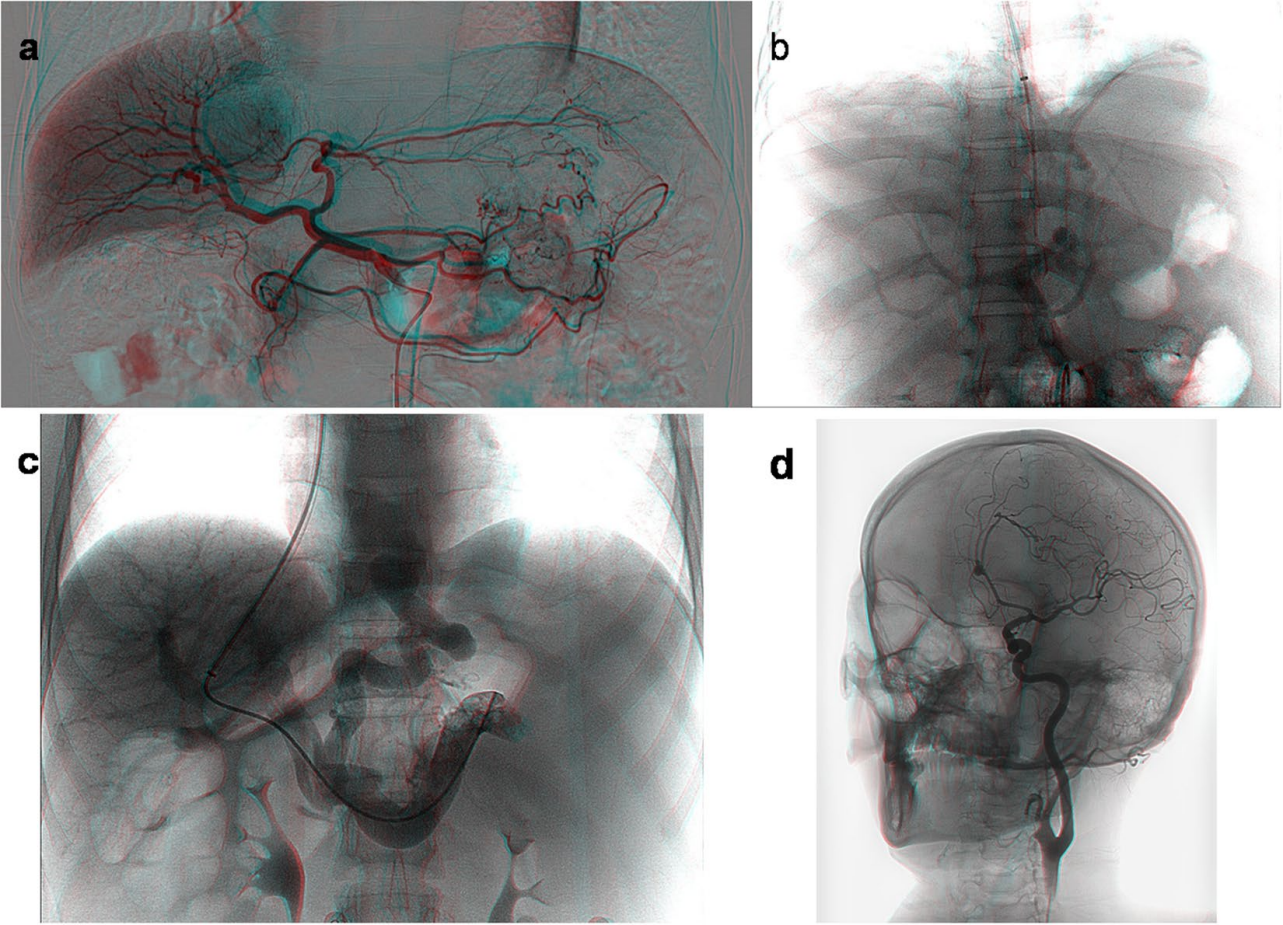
The above duplicated X-ray images (in Fig. 2) of angiography were translated into red-blue 3D images. Stereo images were viewed with a pair of red-blue 3D glasses (a–d).
